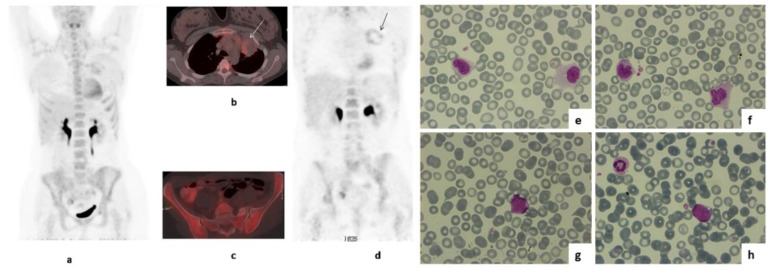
MIP (a) axial-fused (b,c) and coronal PET images (d) of ^18^FDG PET/CT performed during the patient’s follow up, 6 months after the completion of treatment with R-DA-EPOCH. There is diffusely increased ^18^FDG uptake by the bone marrow with intensity higher than that of the liver (a,c,d). As no Granulocyte Colony Stimulating Factor (G-CSF) was administered, this was a new finding. Increased peripheral, “ring like” ^18^FDG uptake by the residual large mediastinal mass was also present with intensity higher than that of the liver—-SUVmax_lesion_:4 versus SUVmax_liver_:2,6 (b white arrow, d black arrow). Peripheral blood smear simultaneously revealed abnormal monocytes (e,f), and blast cells appeared one month later, when the evolution of t-MDS into acute myeloid leukemia was demonstrated (g,h).
